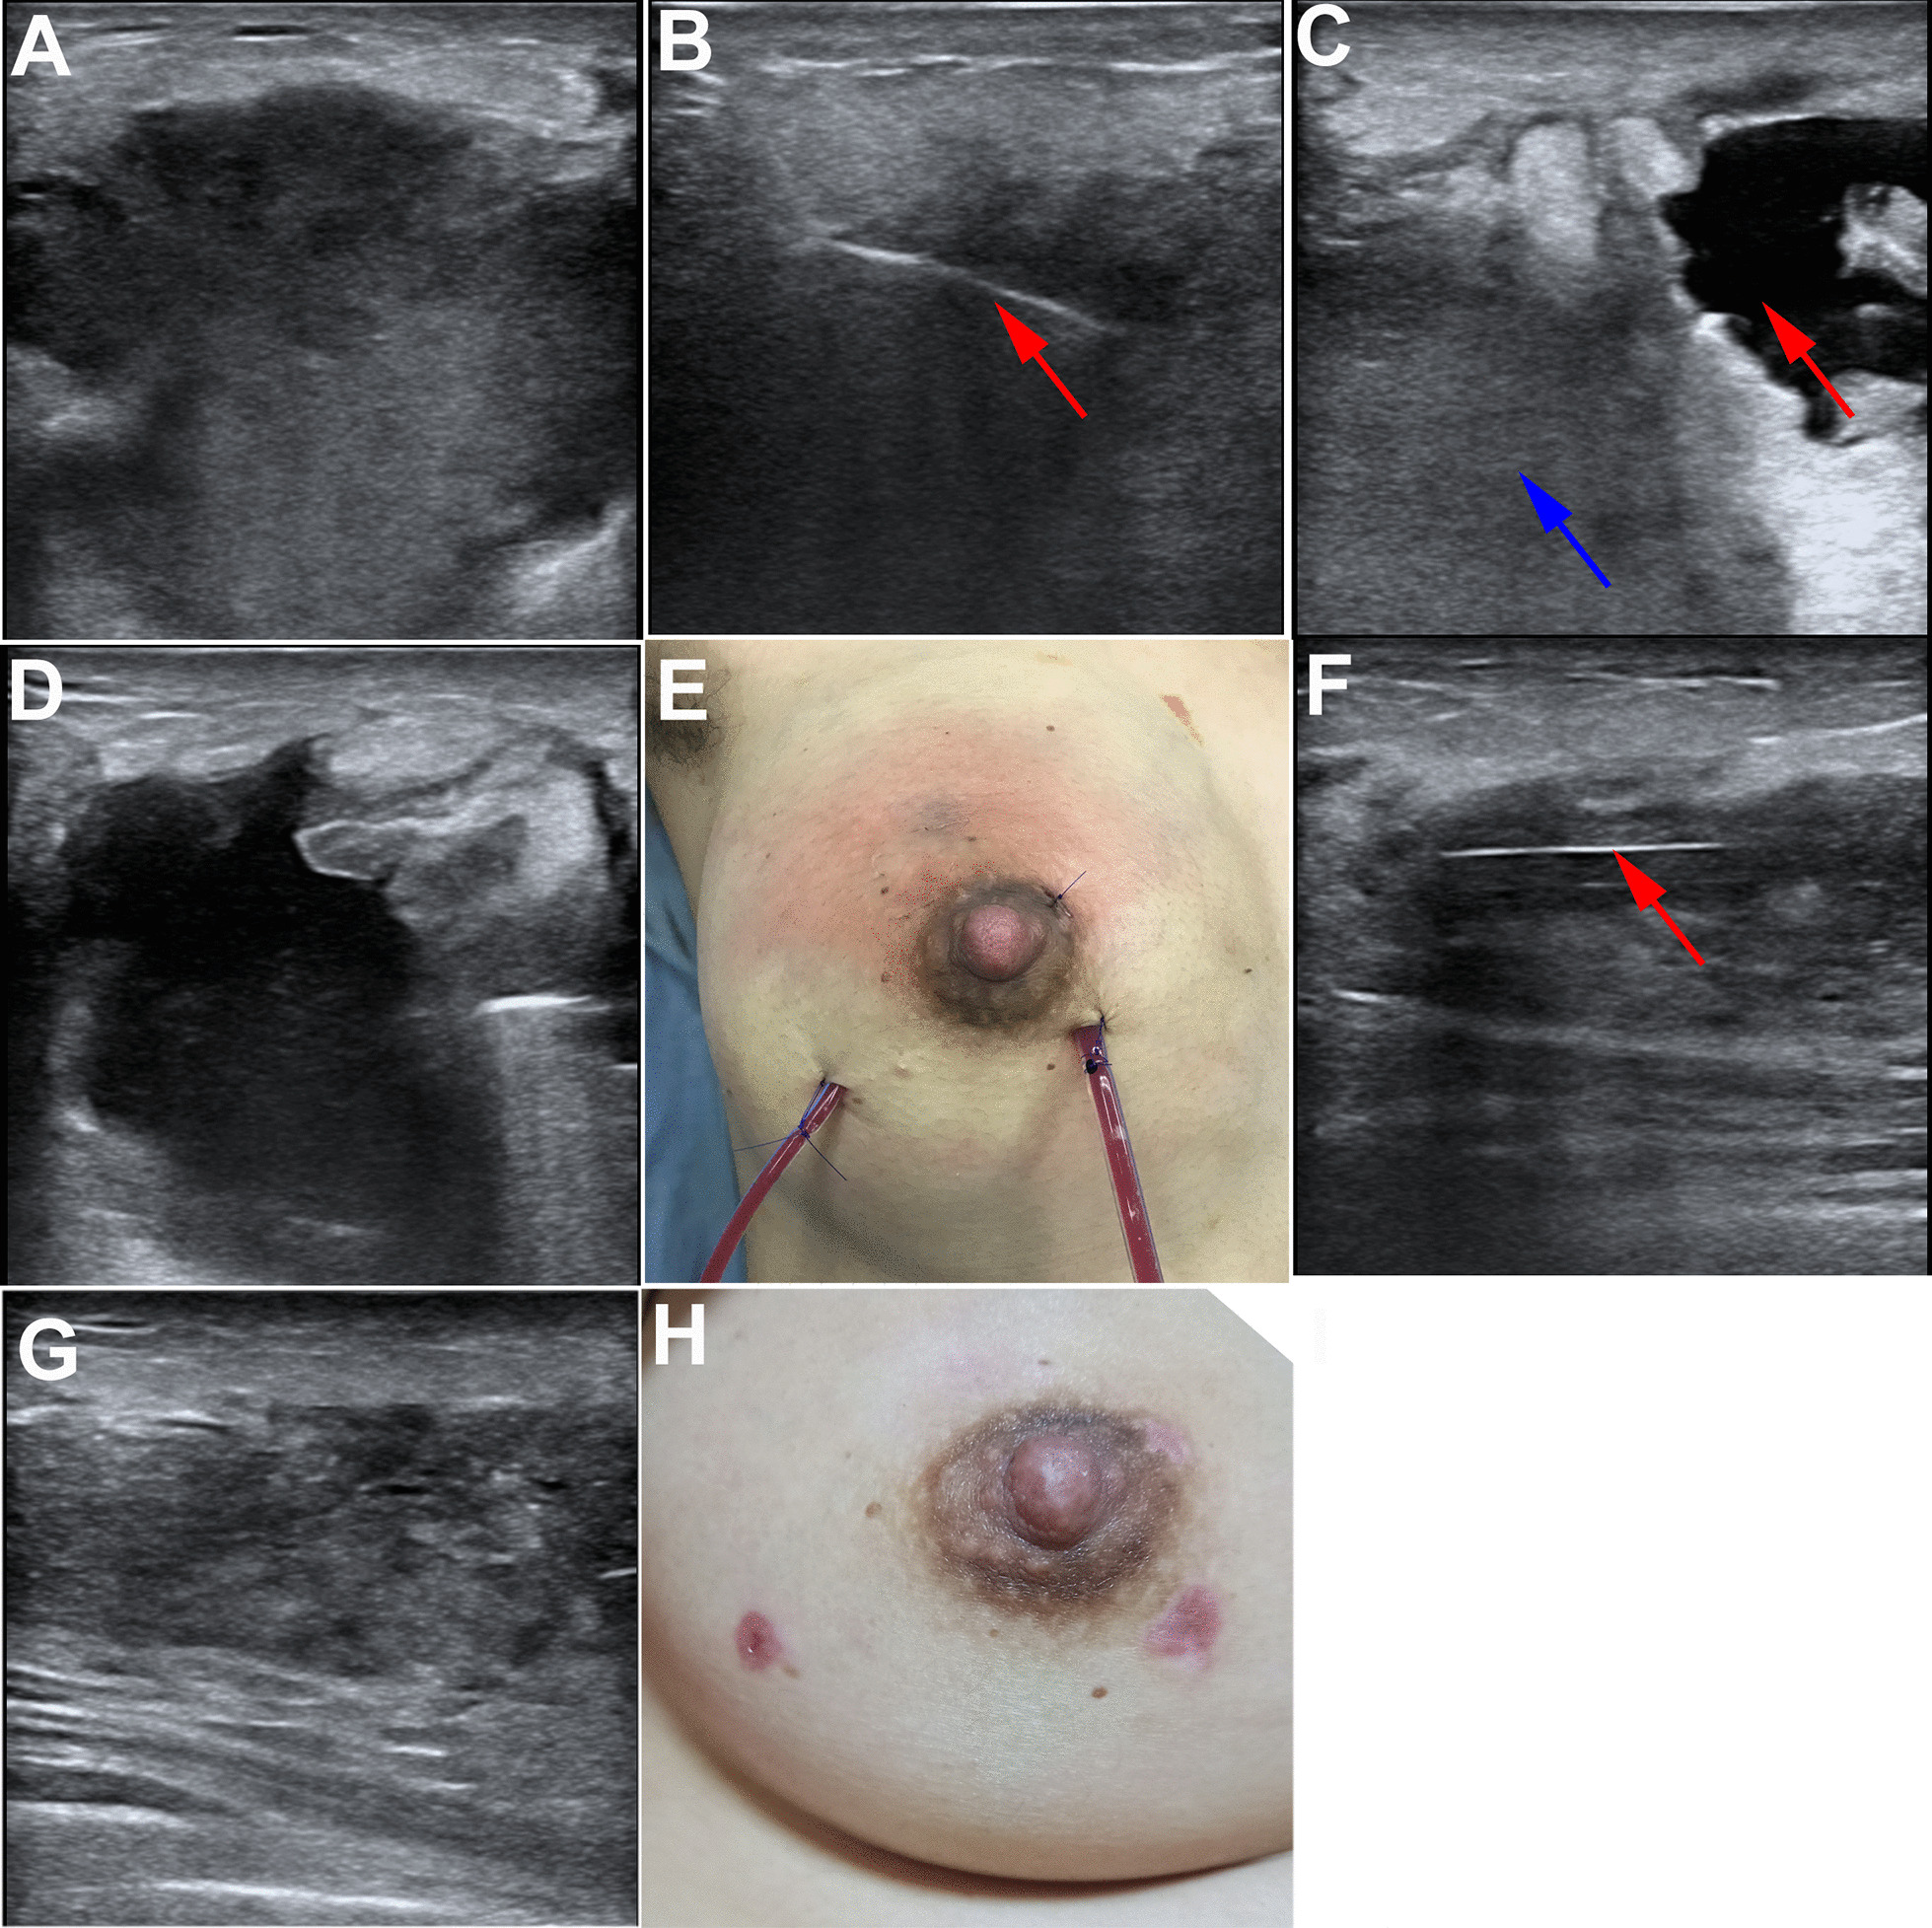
Ultrasound-assisted throughout the management. A Preoperative ultrasonography was used to locate the abscess cavity; B Ultrasound-guided placement of the arthroscopic sheath (red arrow); C The abscess cavity (red arrow) and its adjacent abscess cavity (blue arrow) was surgically cleared (red arrow); D The septum between the abscess cavities was opened under ultrasound guidance; E One or two drainage tubes was placed for continuous drainage; F Ultrasound examination on the first day after surgery showed the drainage tube in the original abscess cavity (red arrow); G Ultrasonography was performed after the drainage tube was removed. H Breast appearance at 2 weeks postoperatively
